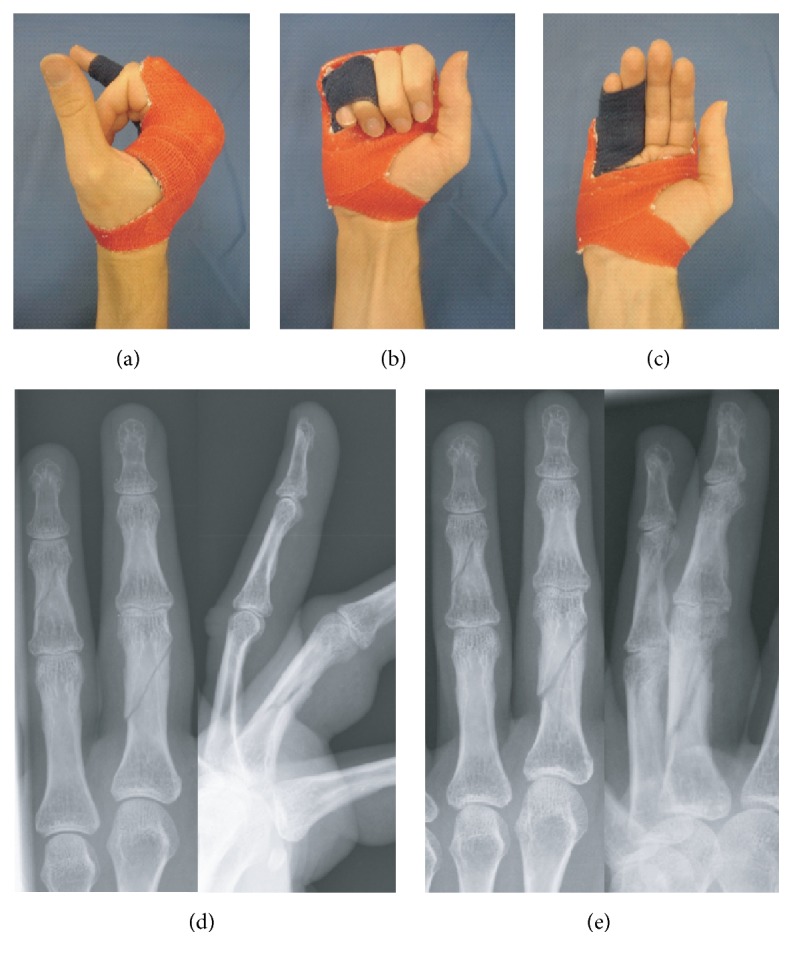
Dynamic finger orthoses with taping: (a) radial, (b) dorsoradial, and (c) palmar view; case sample: nondisplaced 4th proximal phalangeal/5th middle phalangeal fracture, (d) X-ray on admission and (e) at 5 weeks (DASH analysis of case series: *n* = 27; score-mean = 33.30 ± 9.99 SD; DASH follow-up interval (weeks): 9.08 ± 2.33 SD).
